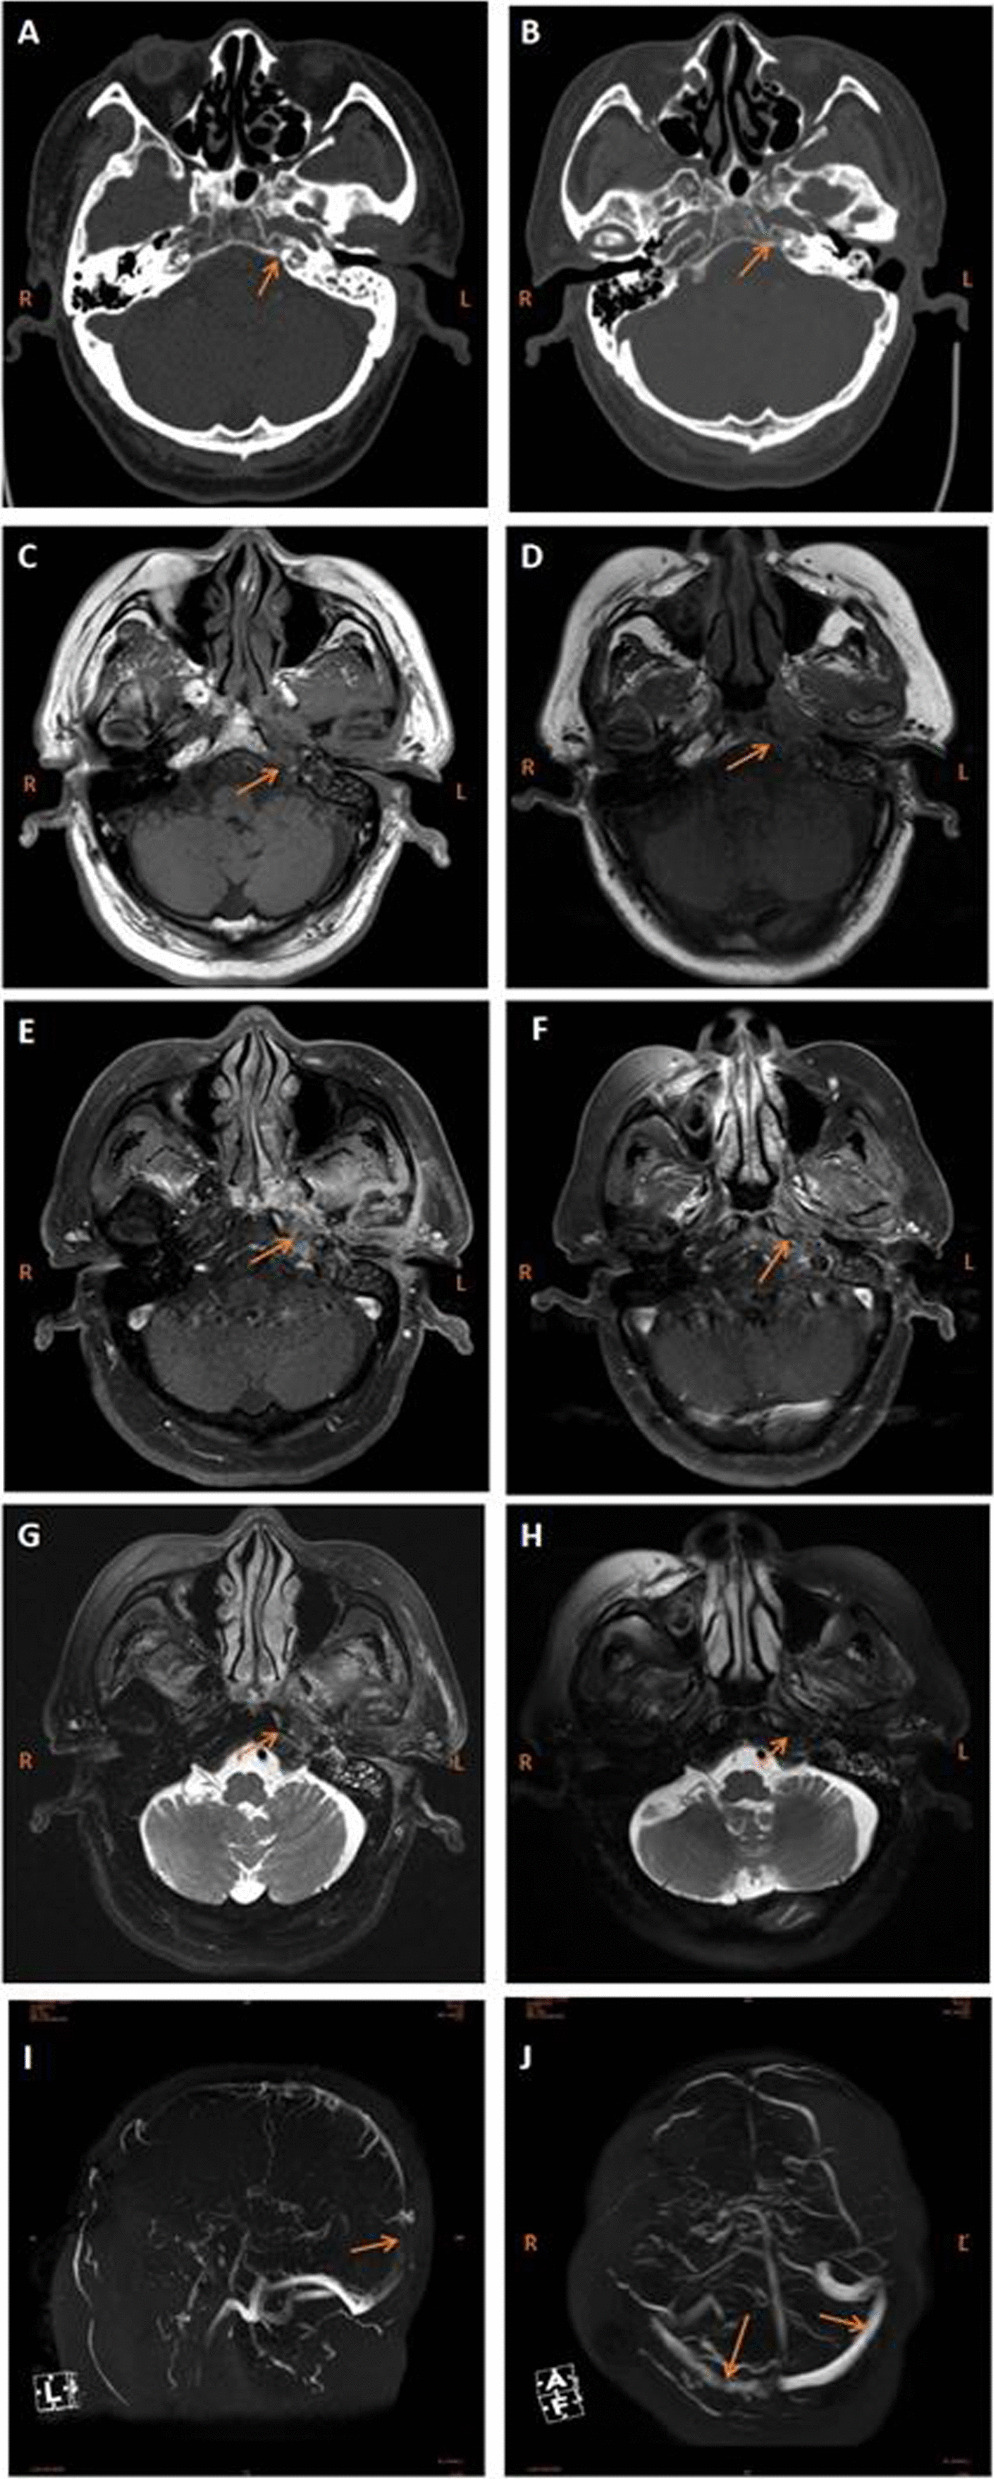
Image of the patient. A and B Pre-and post-operative CT scan images of the middle ear, showing slightly blurred bone in the apical region. C and D Pre-and post-operative middle ear transverse position T1WI MRI, showing low signal in the apex of petrous part. E and F Pre-and post-operative middle ear transverse position T1WI + FS enhancement MRI, showing moderate enhancement in the apex of petrous part, with no significant preoperative or postoperative changes. G and H Pre-and post-operative middle ear transverse position T1WI + FS MRI, showing slightly higher signal in the apex of petrous part with blurred boundaries. I and J Cranial MRV, showing irregular wall of posterior superior sagittal sinus, narrow lumen, low blood flow signal, considerd thrombosis; Left sigmoid sinus normal, Decreased blood flow signal in the right sigmoid sinus
